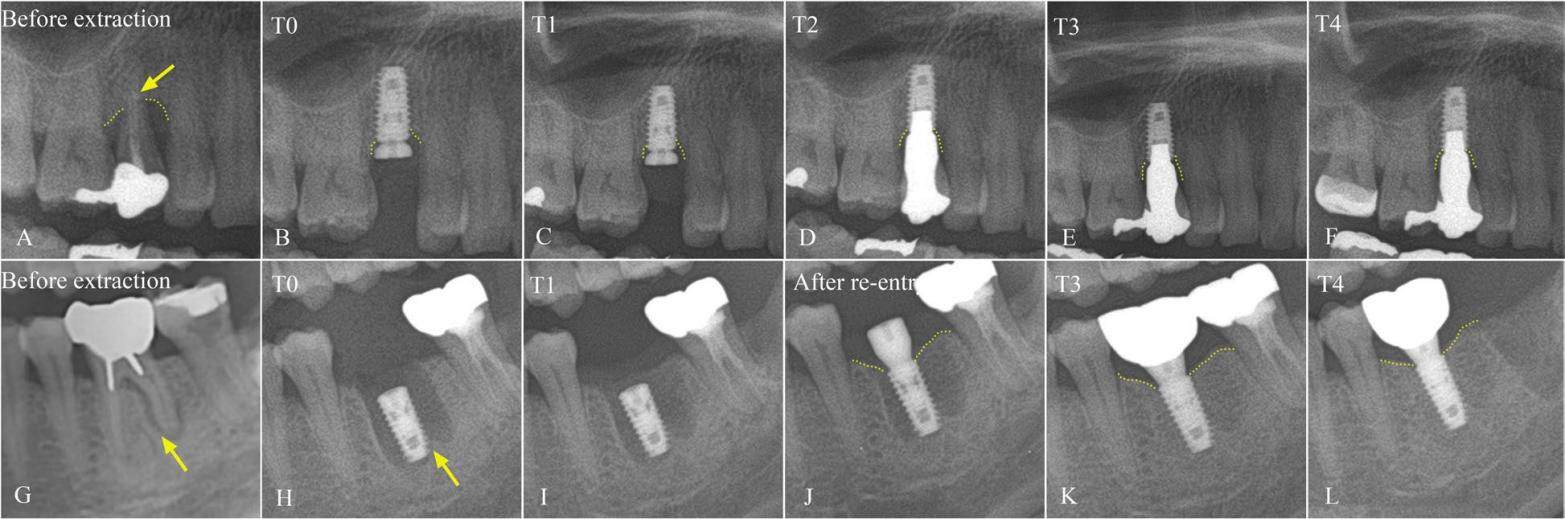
Representative cases of radiographic marginal bone level at maxillary premolar (A–F) and mandibular molar (G–L). Panoramic radiograph before extraction of #15 with radiographic bone loss due to chronic periodontitis (A). Panoramic radiograph taken immediately after immediate implant installation with 4.5 × 8.5 mm Luna® bone level implant (B). Panoramic radiograph at 3 months after implant installation with stable bone level (yellow dots) (C). Panoramic radiographs at 3 months and 1 year after prosthesis loading (D, E) and 5 years after implant installation (F) showing stable and insignificantly increased marginal bone level. Panoramic radiograph before extraction of #36 with periodontal ligament space widening due to periodontitis on the distal root (yellow arrow) with sufficient interradicular bone for immediate implant placement (G). Panoramic radiograph after immediate installation of 4.5 × 8.5 mm Luna® implant in the interradicular bone (yellow arrow) (H). Panoramic radiograph 3 months after implant installation with increased radiographic bone density around implant (I). Panoramic radiographs after re-entry procedure (6 months after initial installation) showing increased marginal bone level around implant platform (yellow dots) (J) and 1 year after prosthesis loading (K) and 5 years after implant installation (L) with stable marginal bone level (yellow dots)
